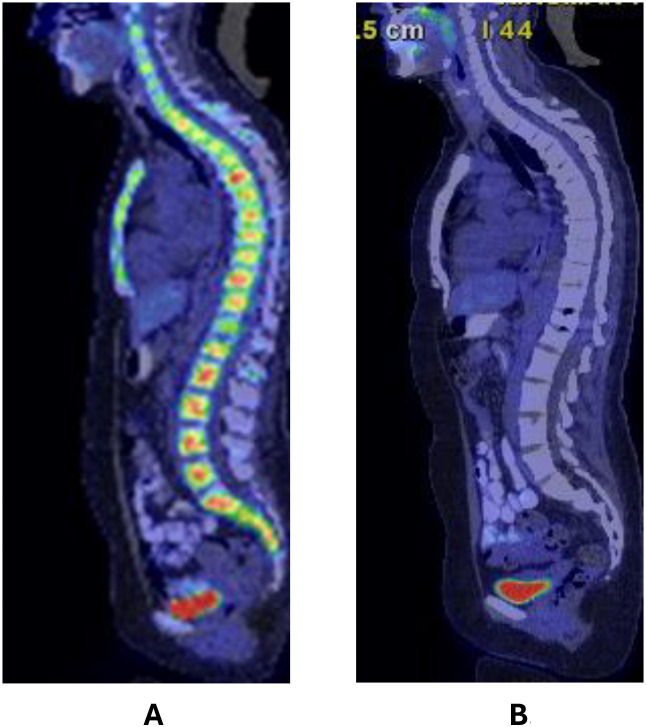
Improvement in tumor deposits in bone with therapy by PET/CT scan. PET/CT before and after treatment. (A) Before treatment (B) 6 months after treatment.
